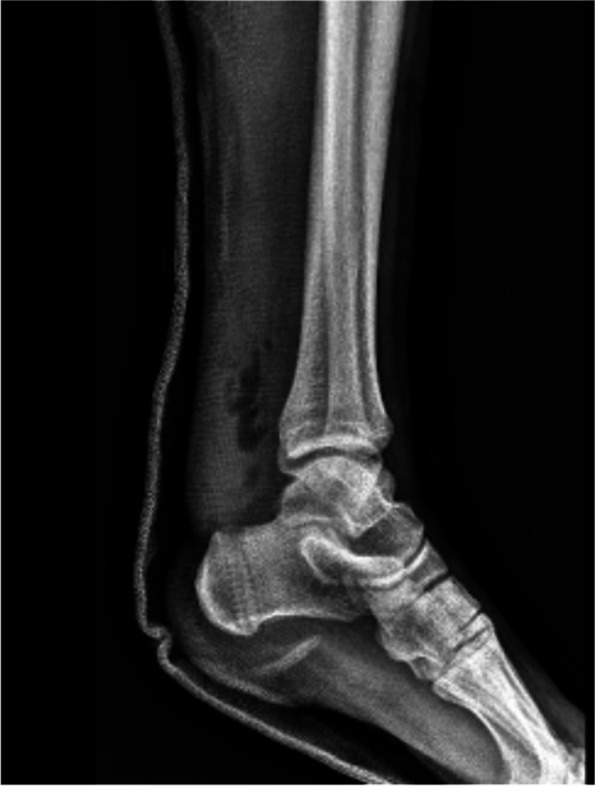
Radiographic control for implant depth assessment in calcaneus anteroposterior and lateral projections
